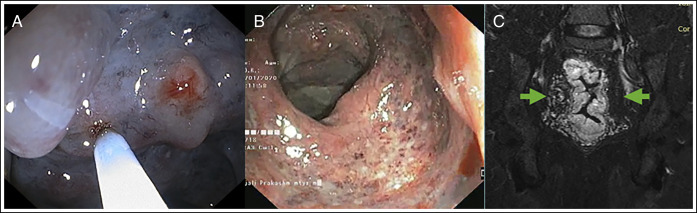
(A) Colonoscopy image showing injection microfoam sclerotherapy. (B) Follow-up colonoscopy image showing remarkable sclerosis of venous malformation. (C) T2-weighted magnetic resonance imaging showing decreased anorectal wall thickening and increased mesorectal fat signal (arrows).
